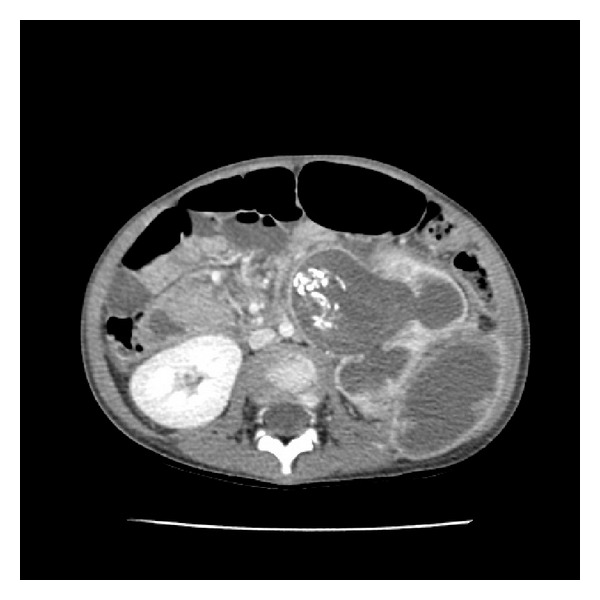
Predrainage CT with contrast demonstrates calcified debris filling the pelvis and proximal ureter. There is destruction of the lower pole renal parenchyma (not pictured) which is contiguous with a 7 × 6 × 5 cm abscess and destruction of the upper pole renal parenchyma with a 4 × 3 × 2 cm abscess.
